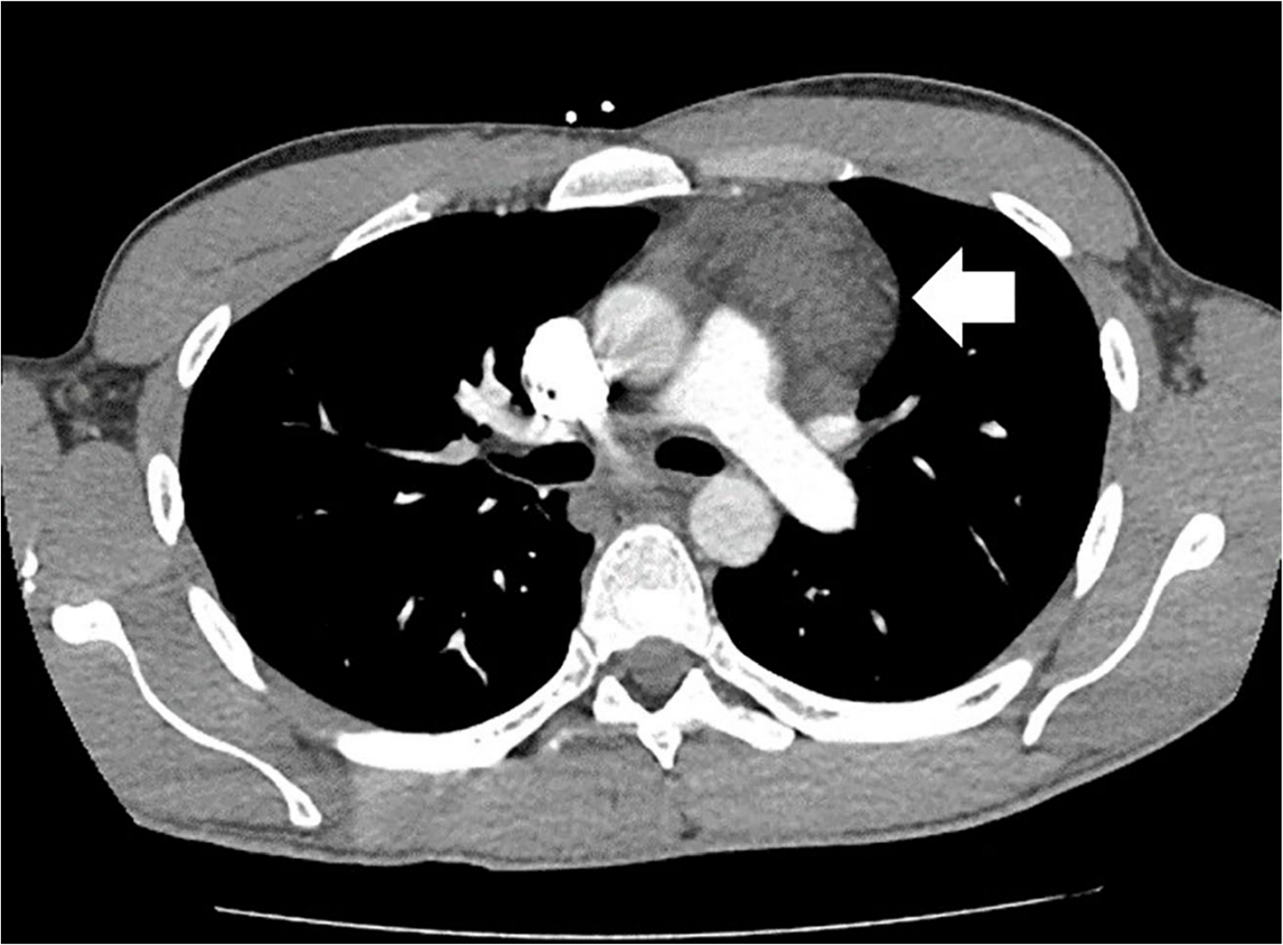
Low density anterior mediastinal mass (white arrow) identified on contrast enhanced CT
